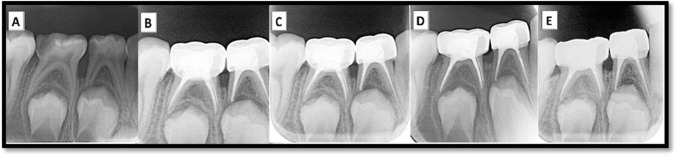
A Preoperative intraoral peri-apical (IOPA) radiograph of lower right second primary molars. B immediate IOPA radiograph post-obturation with ZOE paste. C–E Post-IOPA radiographs at 3, 6, and 12 months
